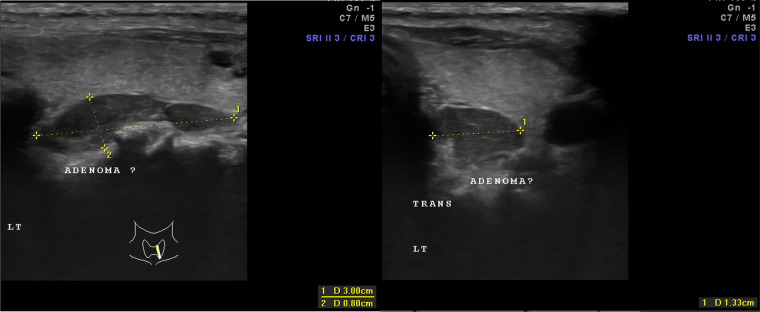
Sonography reports: in proximity to the posterior surface of the mid-portion of the left thyroid lobe, a well-circumscribed, flattened, hypoechoic, solid lesion was identified, measuring 30 × 8 × 13 mm. The mass exhibited significant peripheral and internal vascularity with a centripetal pattern, supplied by a vascular branch originating from the lower pole. Based on these imaging characteristics, the findings were primarily suggestive of a parathyroid adenoma.
